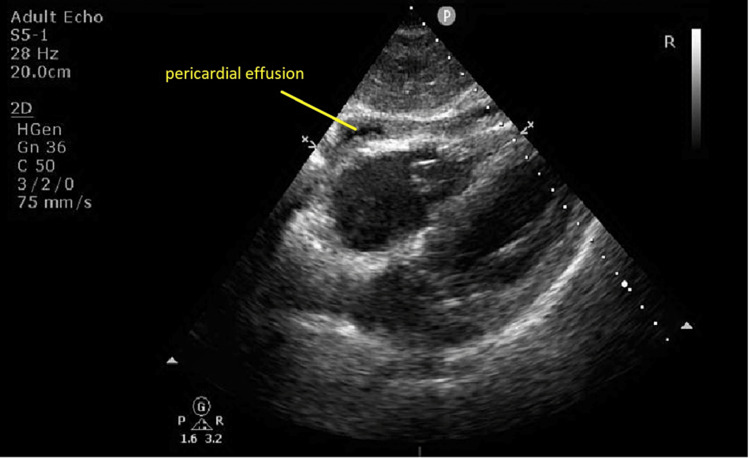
Transthoracic echocardiography: subcostal view Subcostal view of echocardiography showing pericardial effusion
